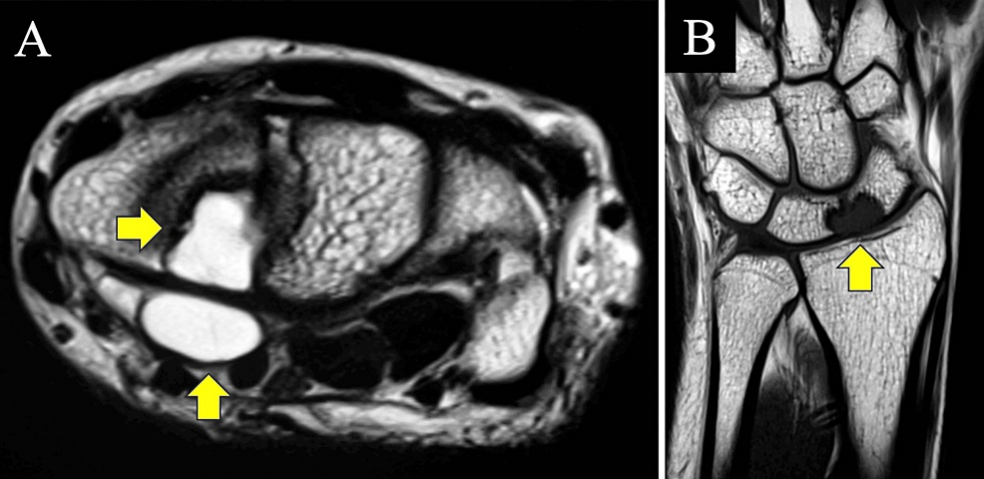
Preoperative magnetic resonance image (MRI) of the left wrist (arrows: ganglion). (A) Axial T2-weighted image of the MRI. (B) T1-weighted coronal image of the MRI.
